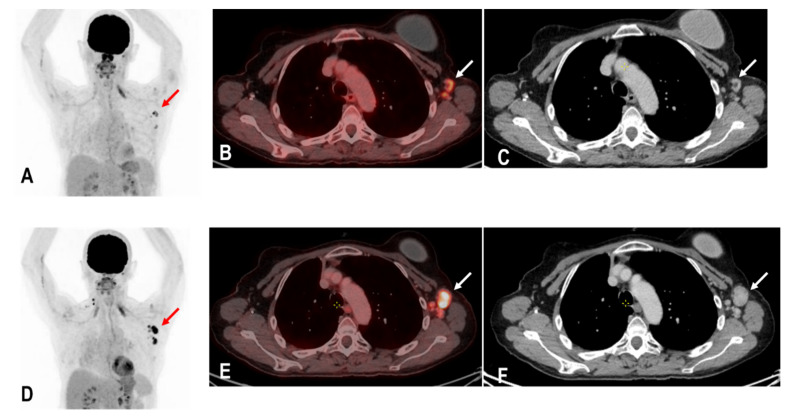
A patient with status post breast carcinoma and hypermetabolic lymph nodes in the ipsilateral lymph nodes of the tumor site (red and white arrows) (A–C). Unfortunately, the patient also received a COVID-19 vaccination on the same ipsilateral side of tumor involvement, and an ^18^F-FDG PET/CT was performed 25 days following the COVID-19 vaccination. Tumor involvement could not be excluded. A follow-up PET/C 3 months later showed a metabolic and morphological progression of the ipsilateral lymph nodes confirming the diagnosis of tumor involvement rather than HLA (red and white arrows) (D–F). Due to known tumor involvement, the patient was excluded from the analysis of this study.
